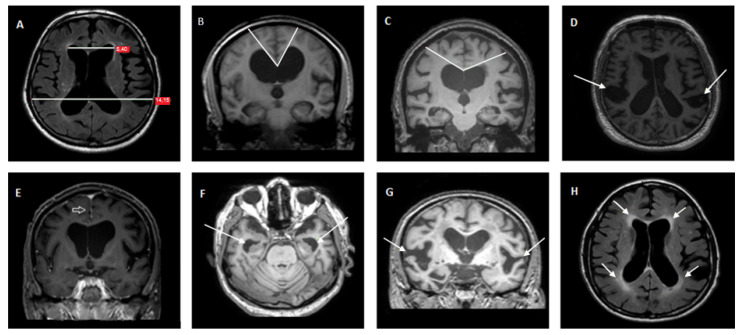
Brain T1-weighted (B-G) and FLAIR (A,H) MRI images of patients of this study. Arrows are pointing to the areas of interest of each imaging parameter. Evan’s Index measurement (graded as 0 if <0.25, 1 if 0.25–0.3 and 2 if >0.3) (A). Callosal angle <60° in a coronal slice (callosal angle is graded as 0 if >90°, 1 if 90° to >60° and 2 if ≤60°) of a tap test responder (B) and >90° in a coronal slice of a non-responder (C). Focally enlarged sulci in a transverse slice (graded as 0 if not present, 1 if present) (D). Narrow sulci in a coronal slice (parameter graded as 0 for normal, 1 for parafalcine and 2 for vertex) (E). Dilated temporal horns in a transverse slice (graded as 0 if <4 mm, 1 if 4 to <6 mm and 2 if ≥6 mm) (F). Dilated Sylvian fissures in a coronal slice (graded as 0 if normal, 2 if enlarged) (G). Diffuse periventricular hyper intensity in a transverse slice (graded as 0 if not present, 1 for frontal horn caps and 2 for confluent areas) (H).
